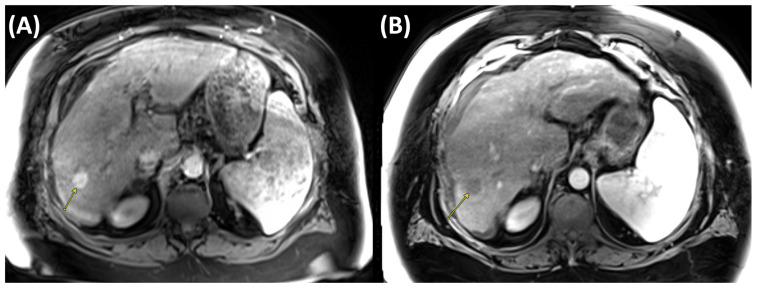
Axial contrast-enhanced MRI of the liver in the arterial phase demonstrating hepatocellular carcinoma (HCC) before and after microwave ablation (MWA). (A) Pre-treatment scan showing a hyperenhancing lesion in the right hepatic lobe measuring 2.0 cm (arrow). (B) Post-ablation scan demonstrating no arterial enhancement within the ablated tumor (arrow).
